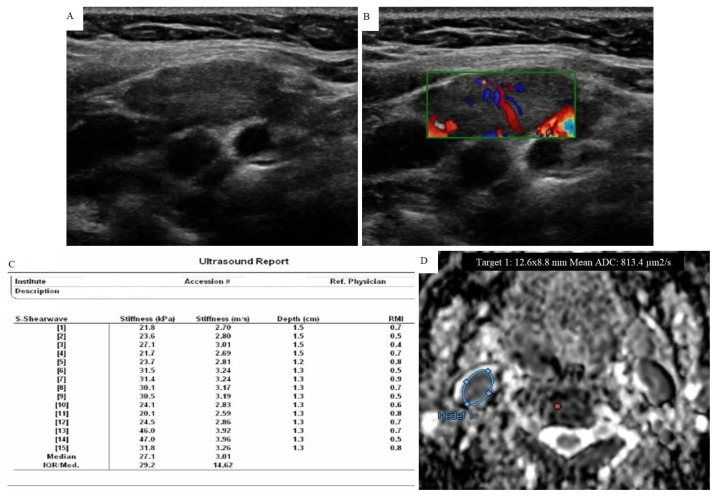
A 48-year-old woman with benign cytology diagnosed with FNAB. A) There was a lymph node that newly developed in follow-up with 13 × 9 mm size, well-circumscribed, smooth border and ovoid-shaped at the level II on the right side of neck, in the case with a history of thyroid papillary cancer on the same side. However, normal fatty hilum could not be identified. B) Doppler US examination showed type II vascularization. C) pSWE parameters are shown in the table. The median stiffness value was found as 27.1 kPa (3.01 m/s) according to the elasticity measurement. D) The ADC map shows the area where the measurement was made. The average ADC value was found to be 0.813 × 10^−3^ mm^2^/s. It was a remarkable example with low ADC value and showed that MRI could be confusing with unnecessary use.
